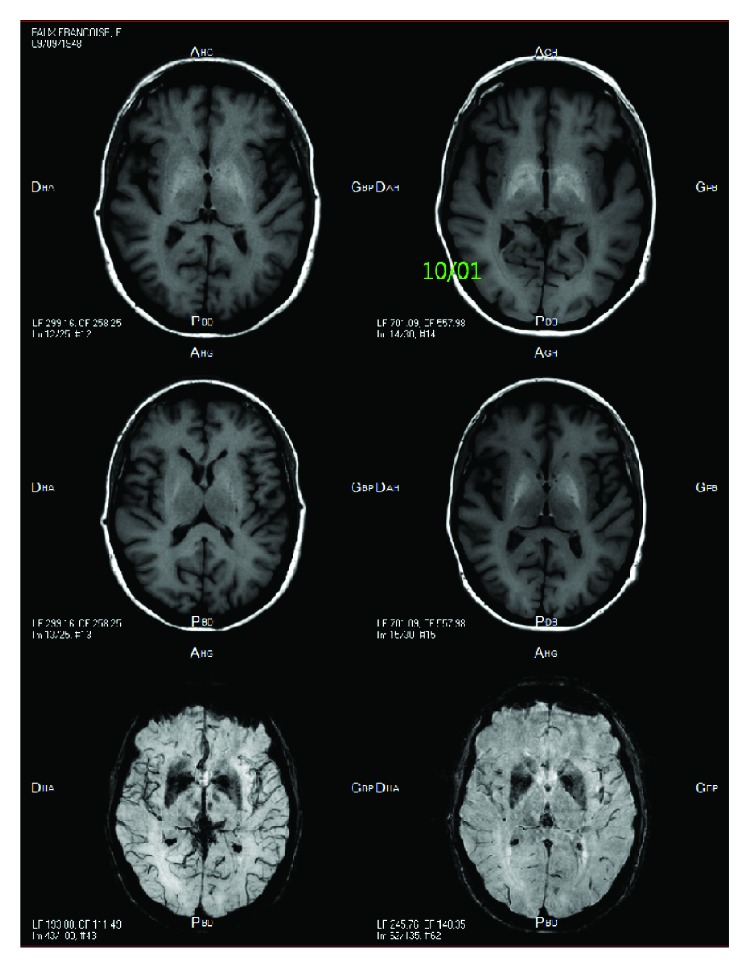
MRI showing the important metal accumulation in the basal ganglia and caudate nucleus with T1 hypersignal and T2 hyposignal.
